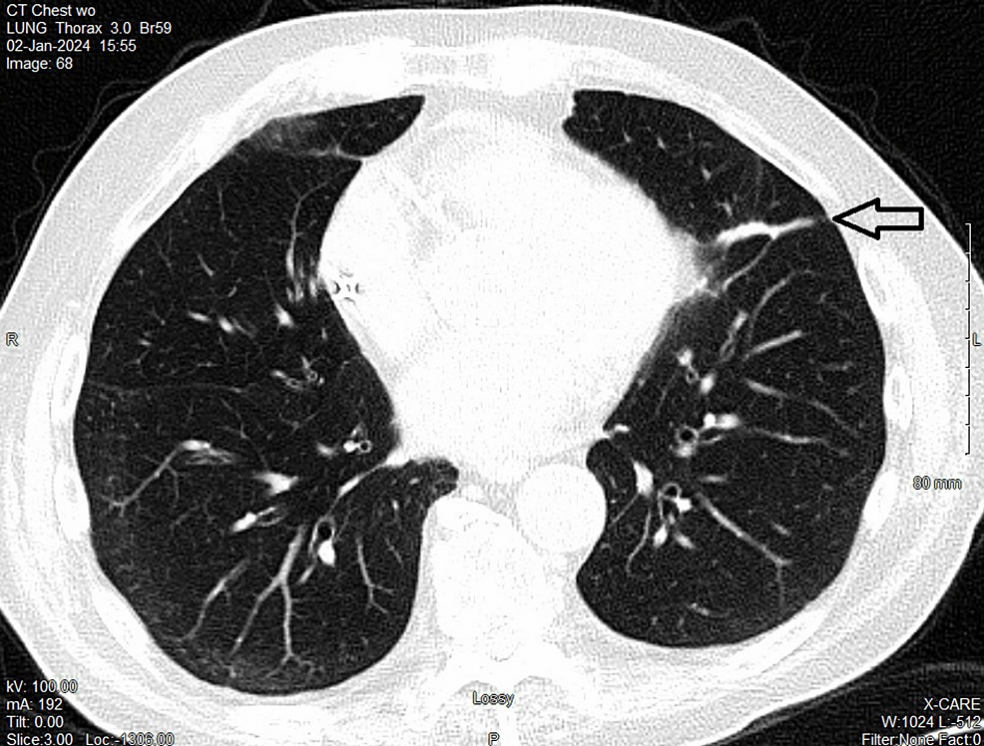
CT in 2024 showing stable scar tissue without recurrence three years after VMAT to the second lung OM CT, computed tomography; OM, oligometastases; VMAT, volumetric modulated arc therapy
